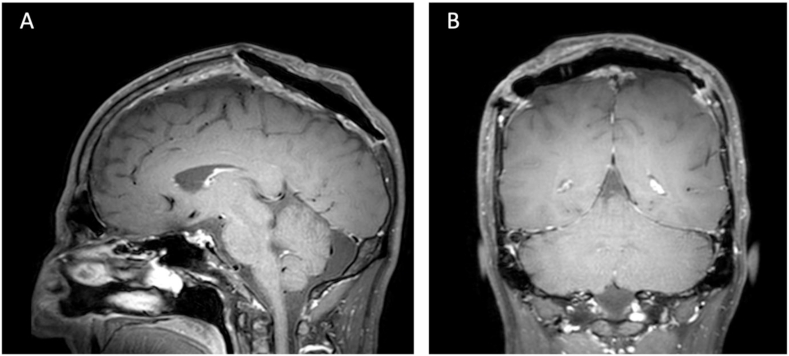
Postoperative MRI imaging following surgical resection. A-B: Postoperative MRI with contrast-enhanced T1-weighted black blood fat suppressed images (sagittal and coronal views) showing no evidence of residual tumor.
